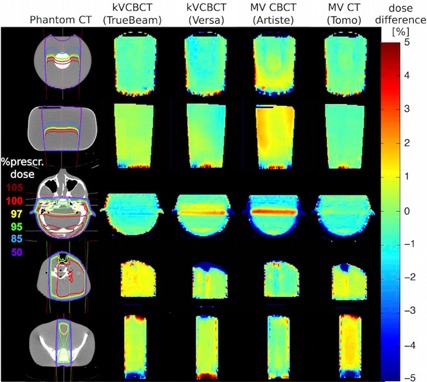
Dose difference maps. Percentage difference of simple dose plans based on kV CBCT, MV CBCT, and MV CT images compared to diagnostic kV CT‐based images. The left column bar shows the dose distribution within the CT image as percentage of the maximum dose. The columns to the right show the relative percentage differences where green is no difference, blue is underdosing, and red is overdosing on the linac's on‐board images.
